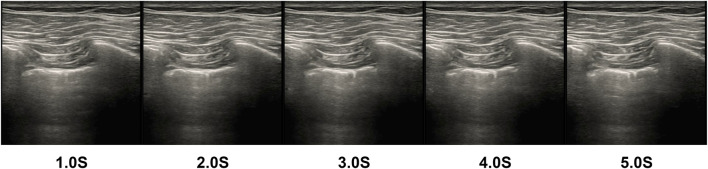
Cases predicted as positive by the model in negative data (Picture frames from the ultrasound video were intercepted at 1-second intervals and stitched together into 1- to 5-second screenshots, with the bright white pleural line in the center of each image almost at rest).
